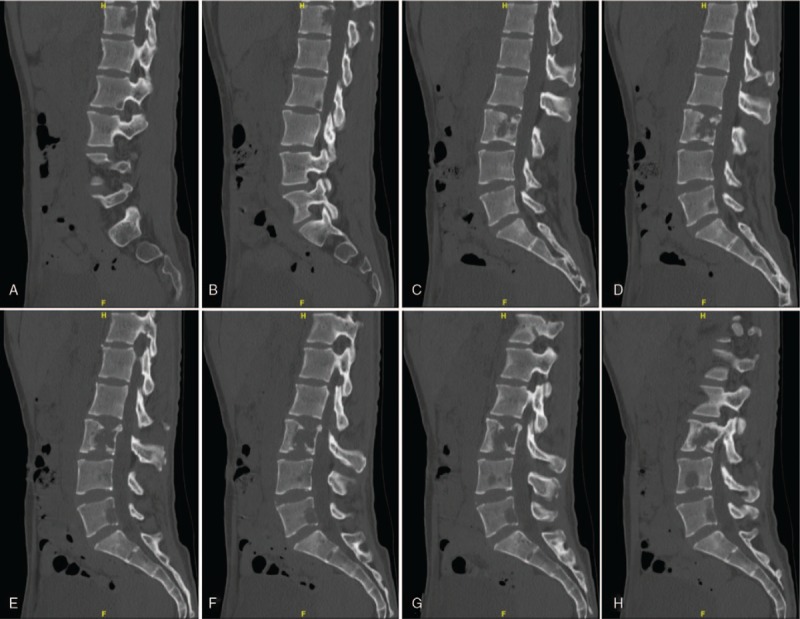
(A–J) Spinal sagittal CT scan revealed obvious bony destruction in the T12 and L3 with intraspinal involvement.
